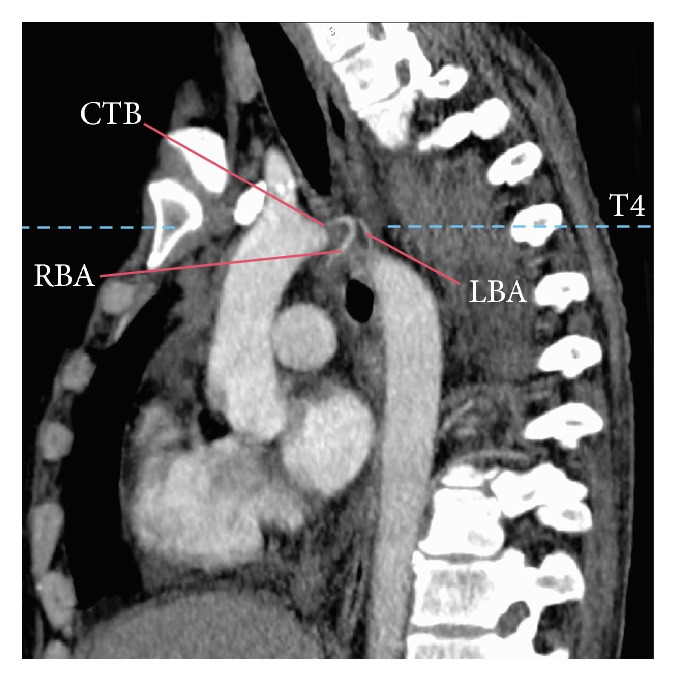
Bronchial artery branching pattern evaluation. A sagittal section of a thoracic CT scan which is used to evaluate the vertebral level of the bronchial artery origin of the bronchial arteries is shown (dashed line). A branching pattern of the bronchial arteries from a common trunk (one right and one left) originating from the aortic arch is also shown. CTB = common trunk of the bronchial arteries, RBA = right bronchial artery, and LBA = left bronchial artery.
